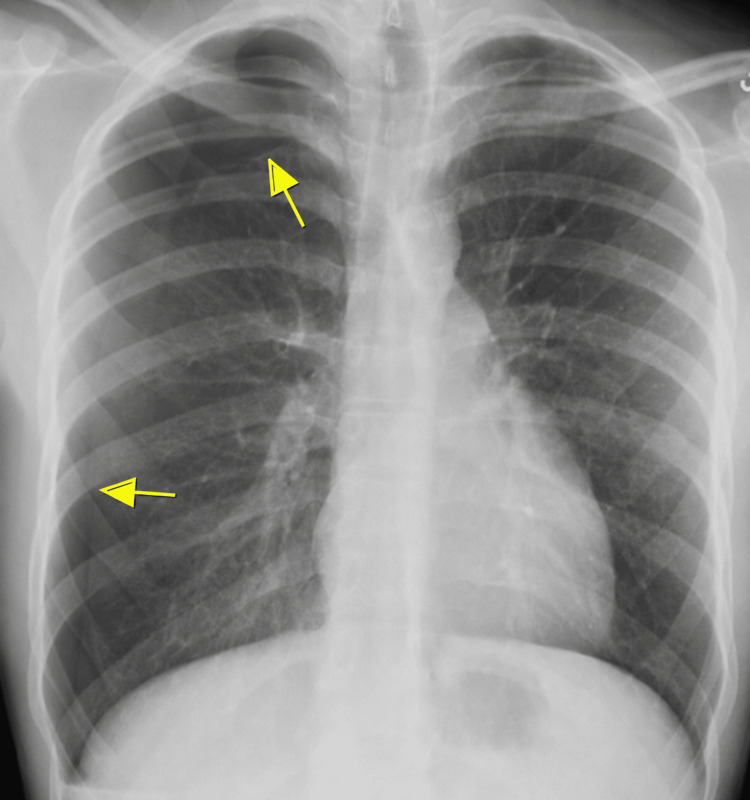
Chest radiograph on presentation showing moderate right-sided PTX (arrows) PTX: pneumothorax
